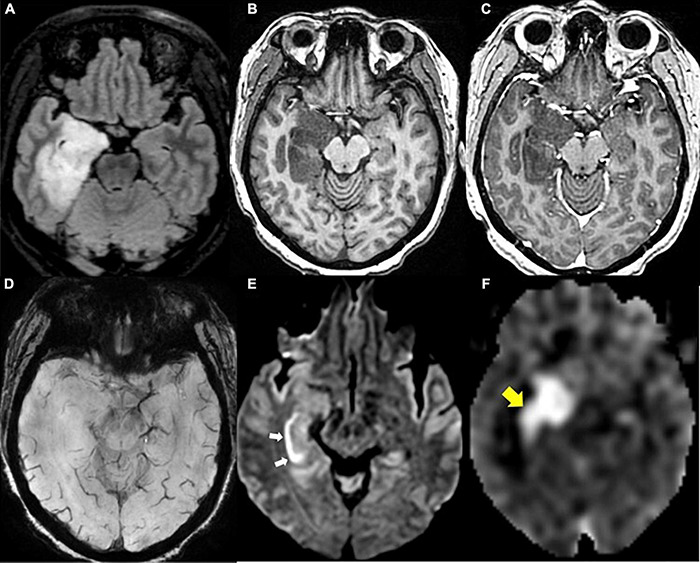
Diffuse glioma, IDH-mutant. (A) Axial FLAIR: Expansile hyperintense mass in the right medial temporal lobe; (B) axial T1 pre-contrast: hypointense mass; (C) axial T1 post-contrast: no associated enhancement within the mass; (D) axial SWI: no blood products or calcium within the mass; (E) axial DWI: linear reduced diffusion (white arrows) in the right hippocampus due to recent seizure activity; (F) axial ASL perfusion: Marked hyperperfusion within the right hippocampus and medial temporal lobe due to recent seizure activity (yellow arrow).
